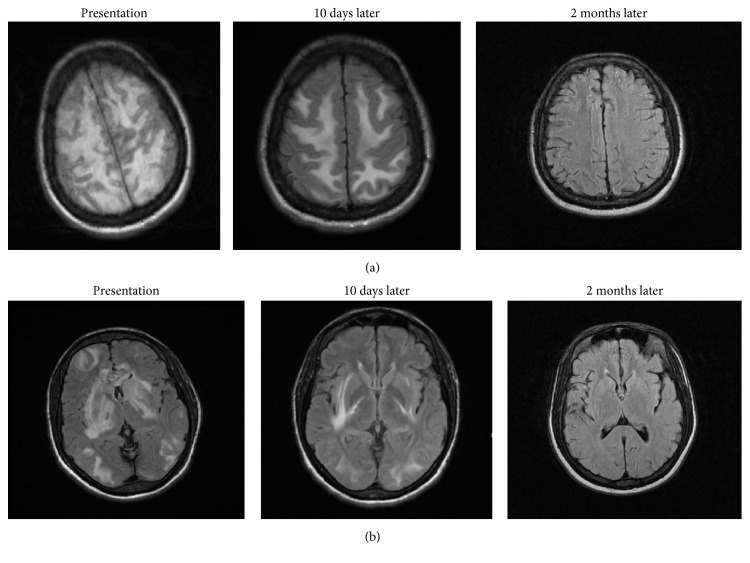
MRI Brain-Axial FLAIR-weighted images demonstrate improvement in the areas of edema over 10 days and 2 months.
